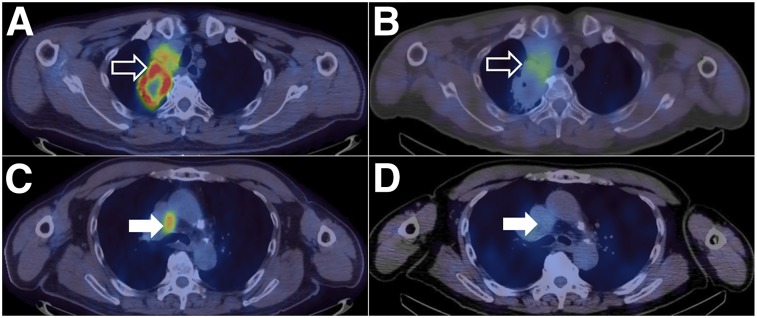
Right upper lobe tumor (open arrows) shows areas of high ^18^F-FDG uptake (SUV_max_ = 16.1) on PET/CT (A) and ^99m^Tc-SPECT/CT (T:BP = 3.53) (B). Mediastinal lymph nodes (closed arrows) show high ^18^F-FDG uptake (SUV_max_ = 6.3) (C) but low ^99m^Tc-NM-01 activity (T:BP = 1.13) (D), demonstrating heterogeneity of PD-L1 expression between primary tumor and nodal sites of disease within same patient.
